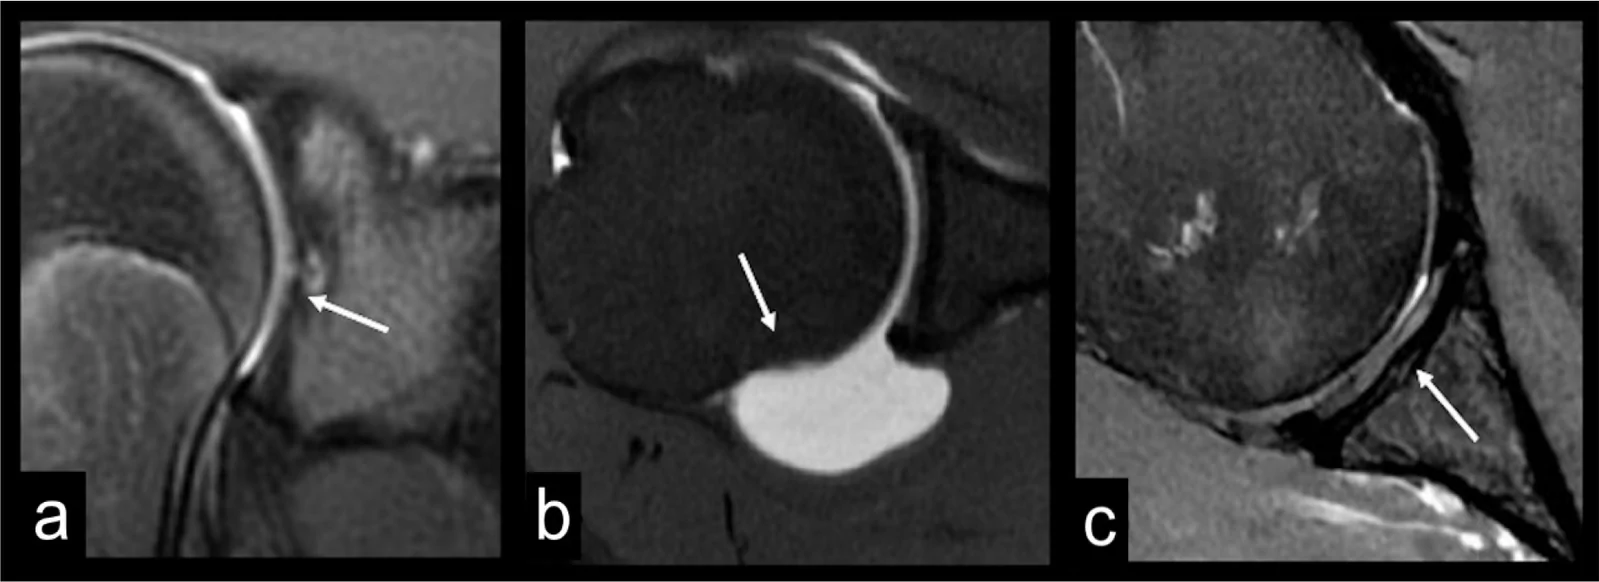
MRI findings of anatomical variants in the glenoid and humeral head.
